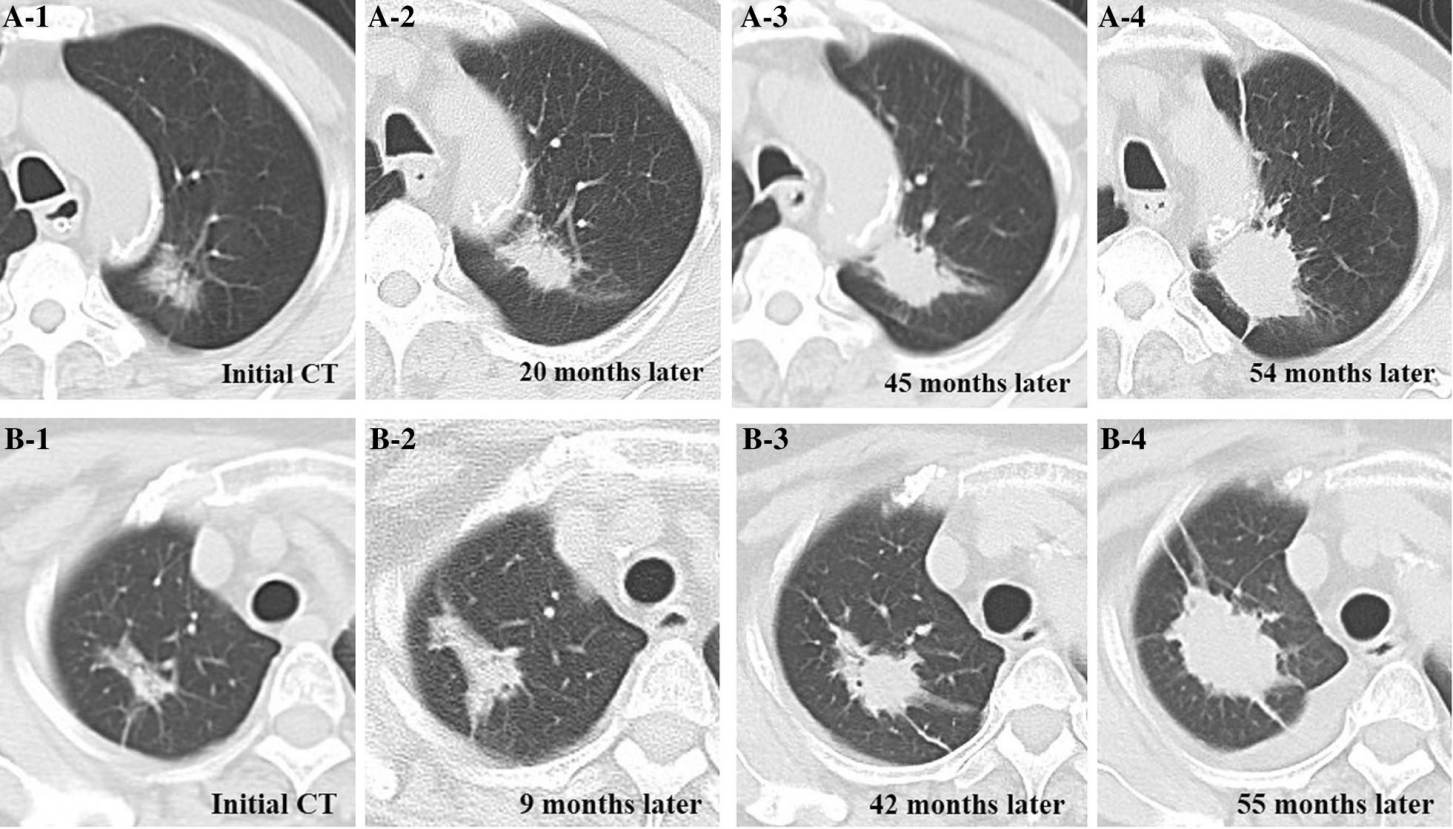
Morphological evolution of L-PLADC during follow-up CT in an 83-year-old man (A) and a 72-year-old woman (B), respectively. A and B The two patients were misdiagnosed as pulmonary inflammatory lesion on initial CT. Sequential CT images of the lung window indicate enlarged focal consolidation, increased attenuation, gradual disappearance of air bronchogram and GGO component; eventually, the focal consolidation became a solid mass during follow-up
